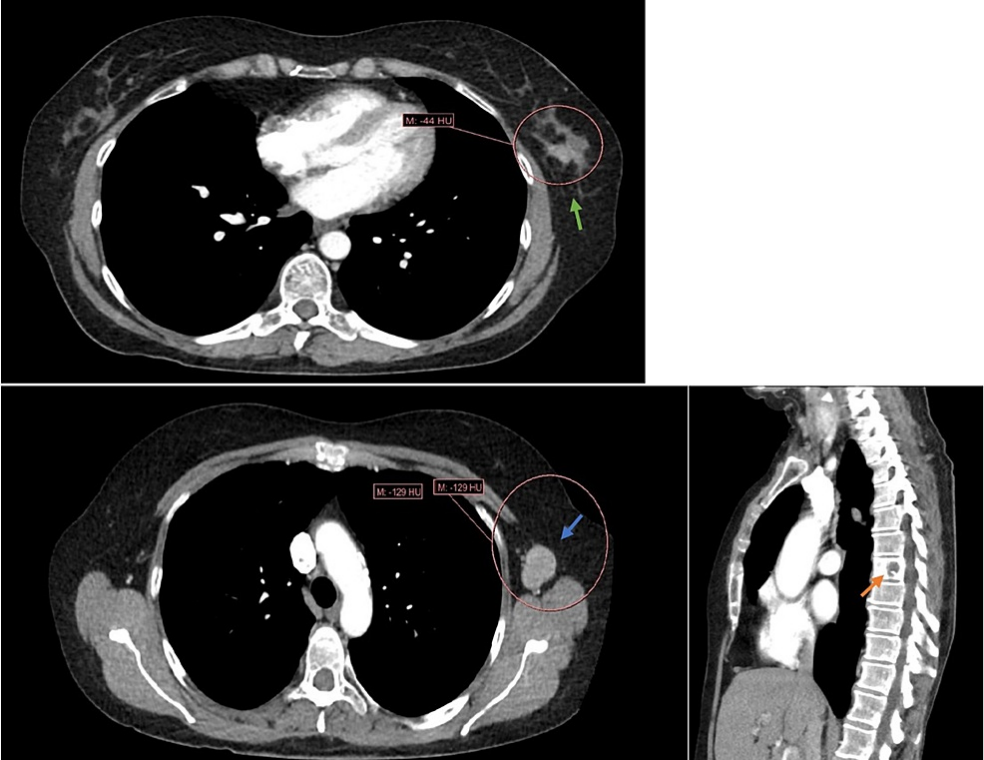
CT scan chest/abdomen/pelvis showing a mass in left breast measuring 2.6 x 2 cm (green arrow), left axillary lymphadenopathy (blue arrow), and a lytic lesion in T7 vertebral body concerning osseous metastasis (orange arrow).
